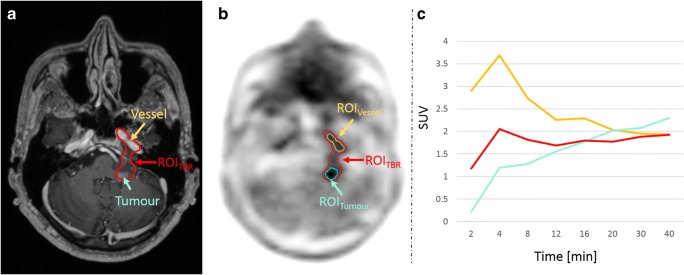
a T1-weighted MRI with a contrast-enhancing lesion (cyan arrow) and the arteria carotis interna (orange arrow). b ROI_TBR_ delineation of a tumour in the PET image after AC using the model-based approach. In this case, the ROI_TBR_ additionally includes parts of the arteria carotis interna. c TACs extracted from ROI_TBR_ (red) and from two manually drawn ROIs in the tumour (ROI_Tumour_, cyan) and the arteria carotis interna (ROI_Vessel_, orange). The TAC extracted from ROI_TBR_ is a mixture of the TAC extracted from ROI_Tumour_ and ROI_Vessel_
